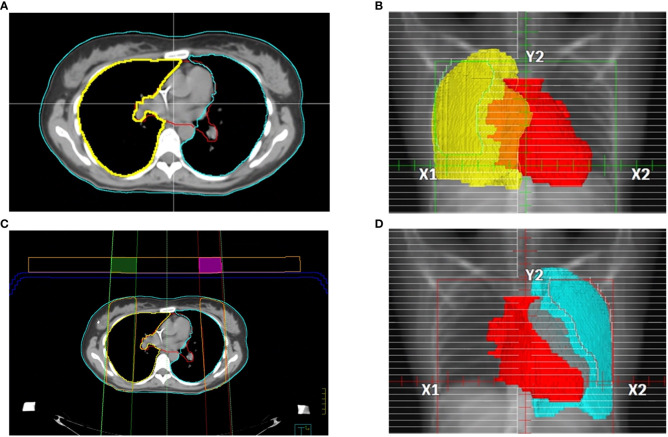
Main steps of lung blocks design: (A) example of axial CT images with several representative contours, in details described in the manuscript. Pinnacle BEV window for (B) right and (D) left lung block design. (C) block tray (in blue) and 3 cm thickness parallelepiped ROI with the right and left block beams, 60% isodose line (in orange). The intersection of the of contours generated by the 60% isodose line and the 3 cm thickness parallelepiped ROI produced the right (green) and left (purple) lung blocks to be 3D-printed.
